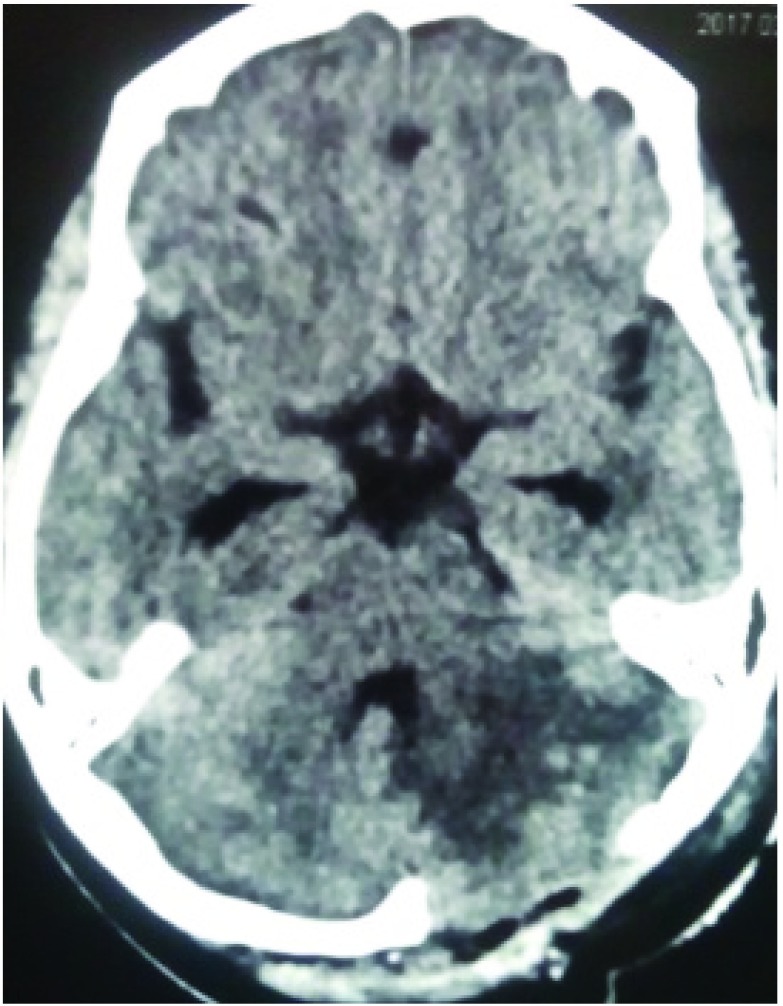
Post-operative CT image showing complete excision of the lesion in the posterior fossa.
